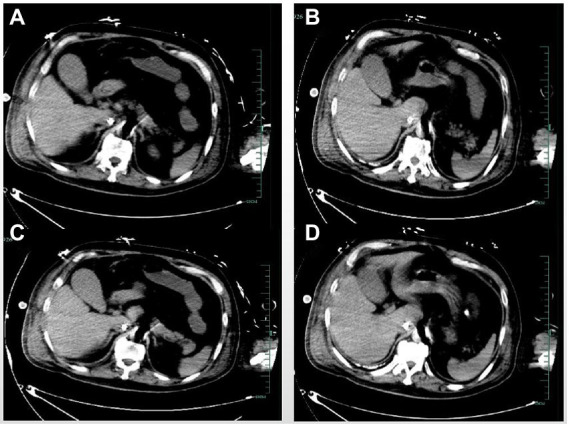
The abdominal computed tomography (A–D) levels showed no significant abnormality of the gallbladder.
